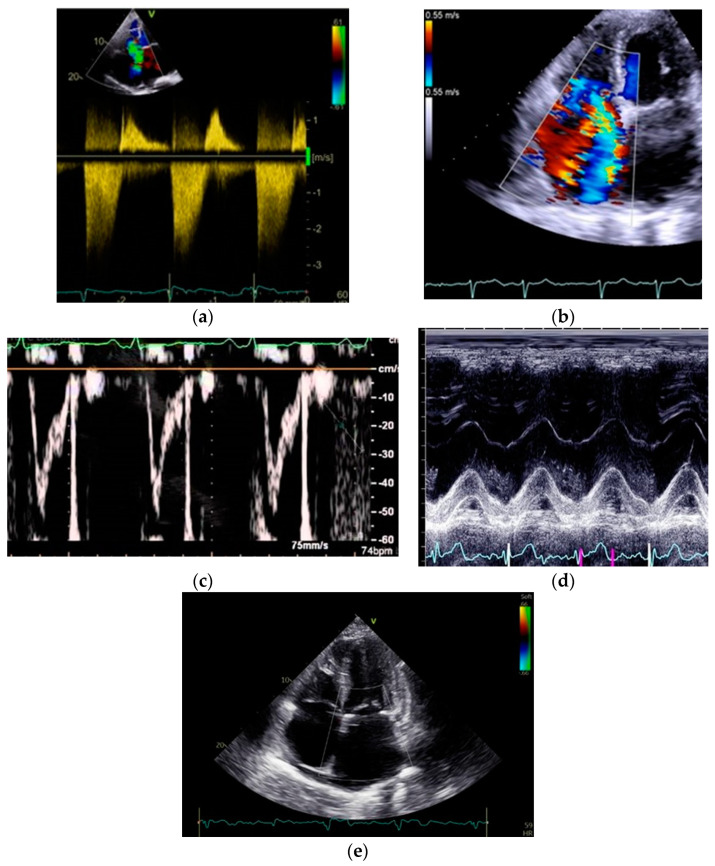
Classical echocardiographic indices of pulmonary hypertension diagnosis and prognosis: (a) TRVpeak doppler; (b) TR color doppler; (c) RVOT-AT; (d) TAPSE; (e) Pericardial effusion and RA enlargement.
